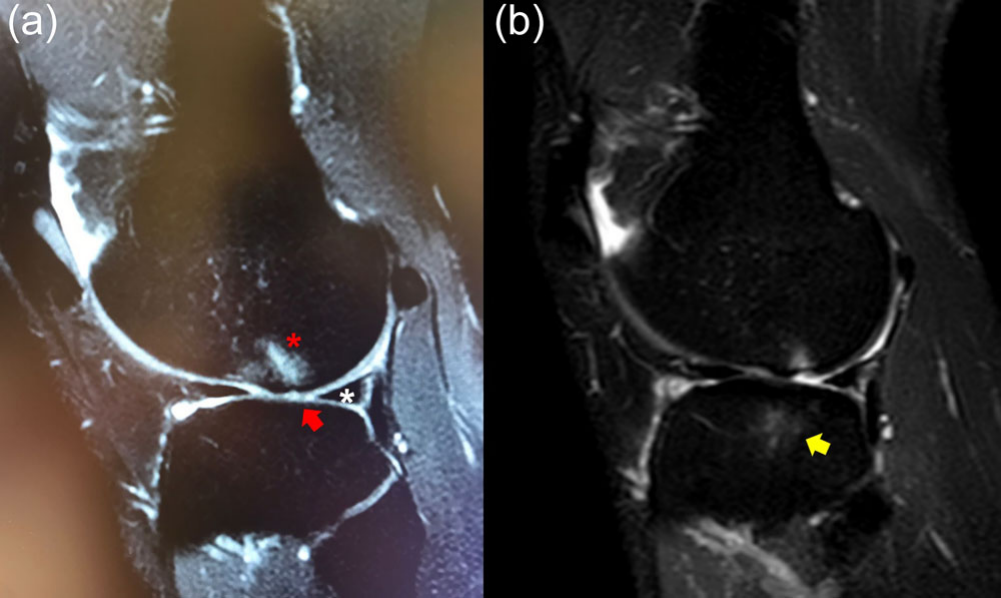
Left knee of a 27‐year‐old patient with a chronic ACL tear. The sagittal MRI of the lateral compartment shows the presence of subchondral bone oedema (red asterisk) and cartilage thinning (red arrow) at the lateral femoral condyle, with an intact lateral meniscus (white asterisk) (a). After 4 years, at the age of 31, the cartilage defect of the lateral femoral condyle was more evident, and bone oedema in the lateral tibial plateau was present as well (yellow arrow) (b). ACL, anterior cruciate ligament; MRI, magnetic resonance imaging.
